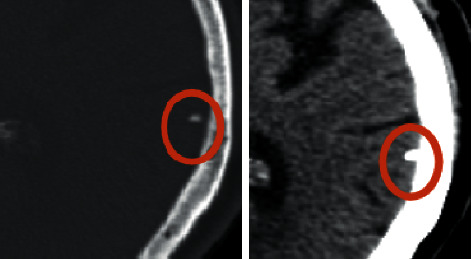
Cerebral CT scan of the index patient.
